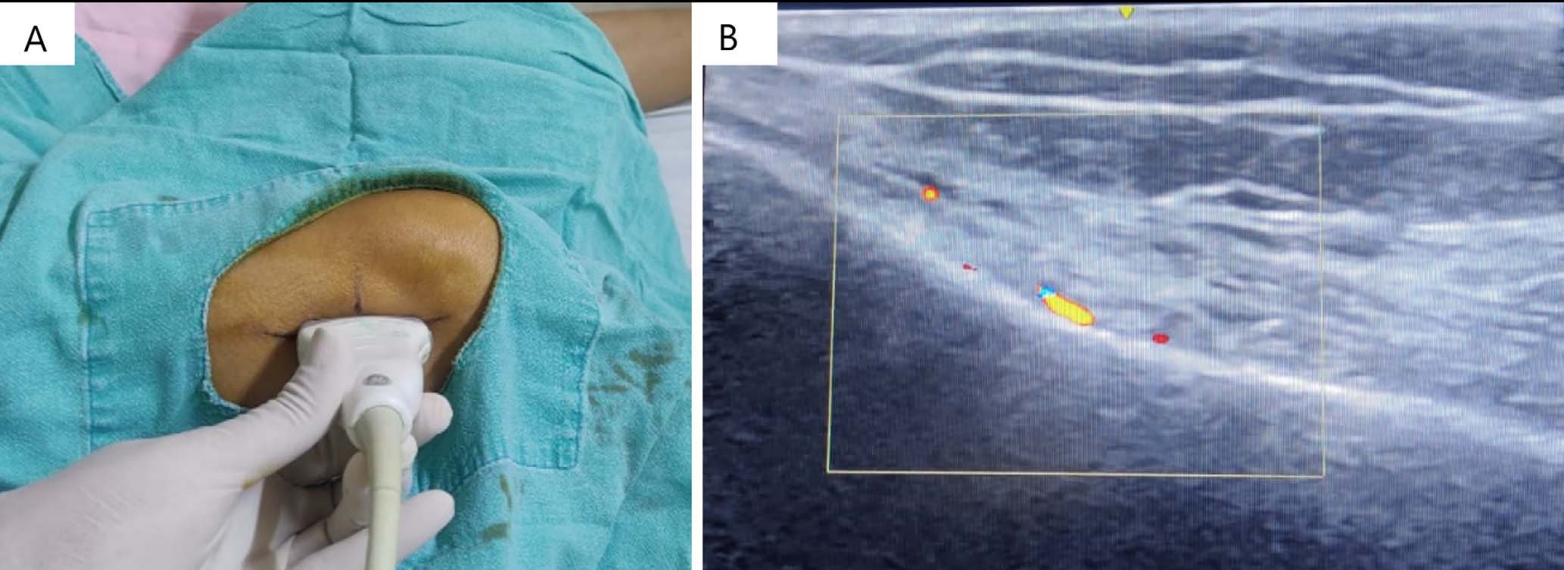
Ultrasonography scan for the right superolateral genicular artery. (A) The ultrasonography scanning is started from the lateral knee joint line, and the ultrasound probe is moved to the metaphyseal-diaphyseal junction of the femur superiorly, looking for the genicular artery overlying the periosteum. Once the genicular artery is visualized, the artery is centralized as per the center point mark on the ultrasonography machine screen. The ultrasound probe's central points are marked at 4 sites: 2 along the longitudinal axis and 2 along the transverse axis of the ultrasound probe. (B) Ultrasonography image with color scan of the superolateral metaphyseal-diaphyseal junction of the femur shows the centralized genicular artery with the center of the ultrasonography screen.
